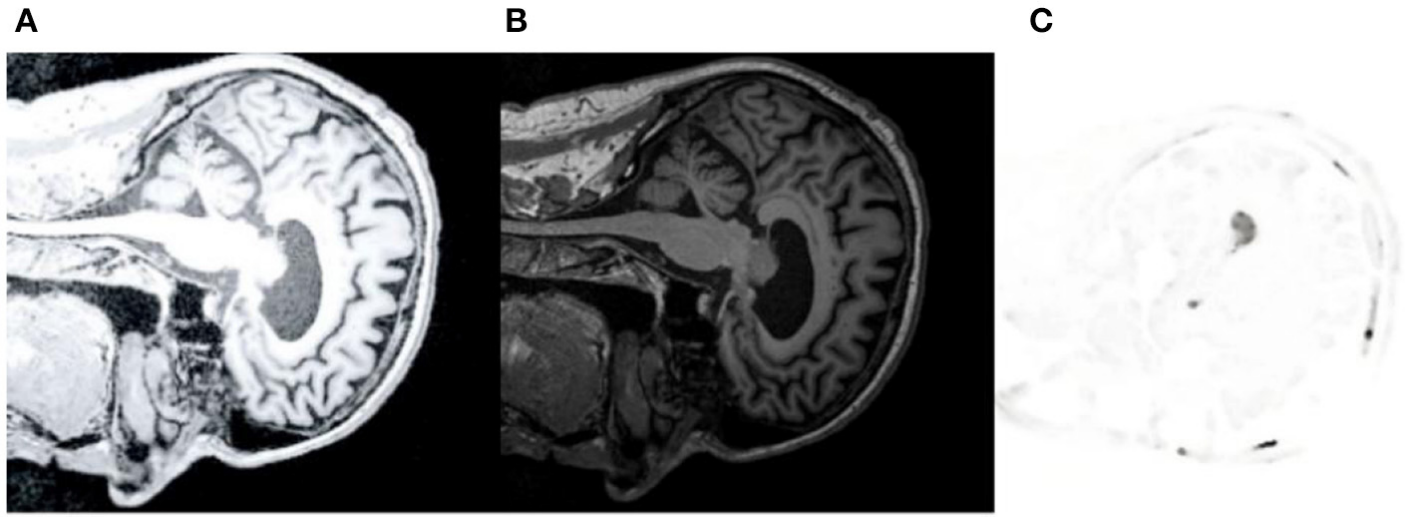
CT/MRI/PET reference image. (A) shows CT reference data images. (B) is MRI reference data image. (C) is PET reference data image.
